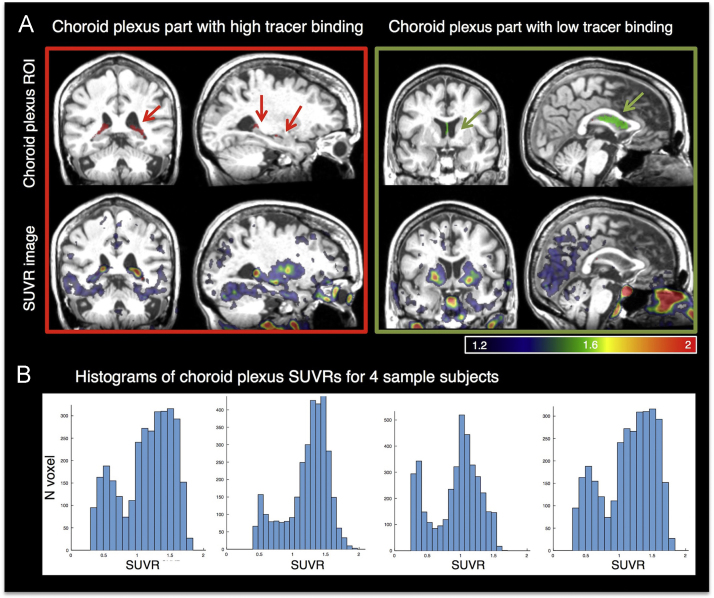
Areas of high (A) and low (B) choroid plexus binding are shown in the masks (top row) and SUVR image (bottom row) for one sample subject. Histograms from 4 subjects show the bimodal distribution of the choroid plexus [^18^F]-AV-1451 binding.
